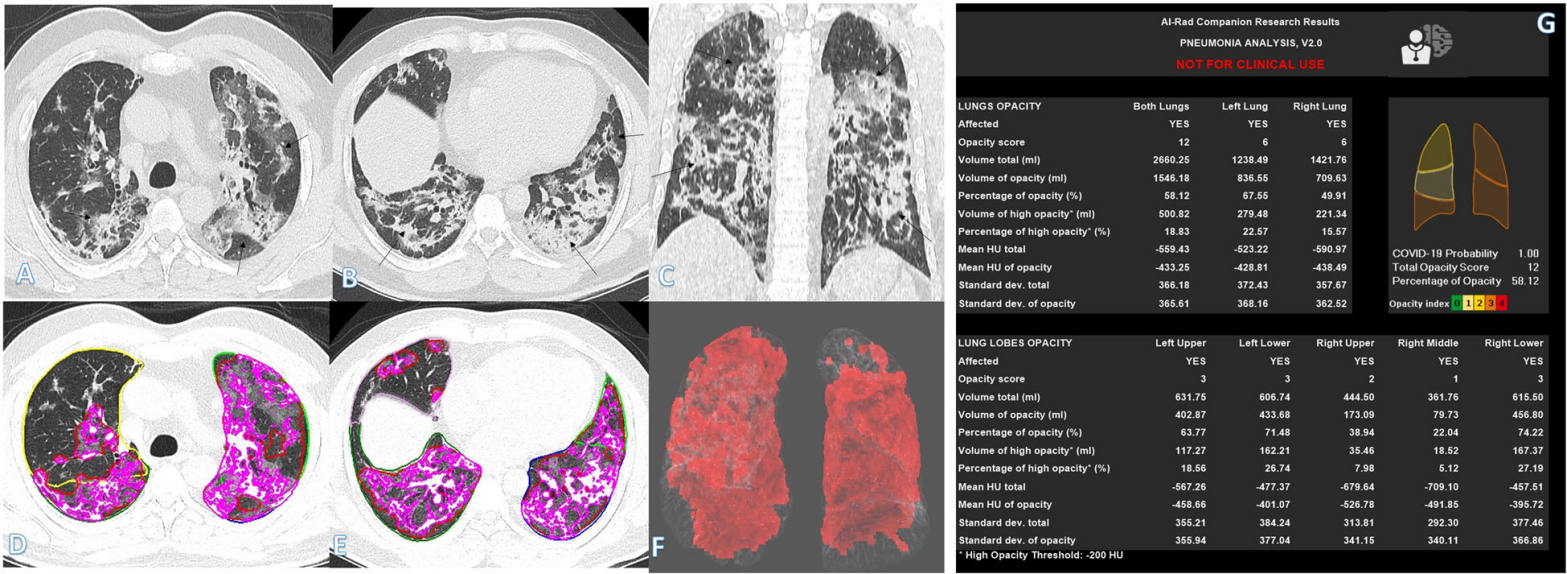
A 46-year-old male with positive COVID-19 virus. CT chest shows diffuse bilateral confluent consolidation with air bronchogram, crazy-paving appearance, subpleural thickening with fibrosis, and organizing pneumonia pattern of COVID-19 (arrows in a–c). Total severity score = 13. Quantitative analysis (d–g) by AI-Rad Companion Research CT Pneumonia Analysis was presented with the measured parameters seen in table (g). Quantitative total opacity score was 12
